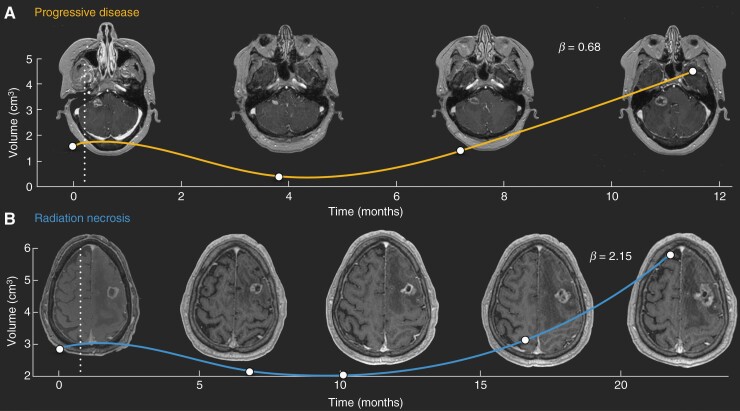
Longitudinal dynamics were observed in (A) Lesion with progressive disease (PD) post-SRS and (B) an irradiated BM diagnosed with radiation necrosis (RN). SRS treatment times are marked with a vertical dashed line. White dots are the measured volumes, and the solid lines are the result of interpolating longitudinal volumetric data (shown only to guide the eye). Axial slices of the contrast-enhanced (CE) T1-weighted magnetic resonance imaging (MRI) sequences are displayed.
